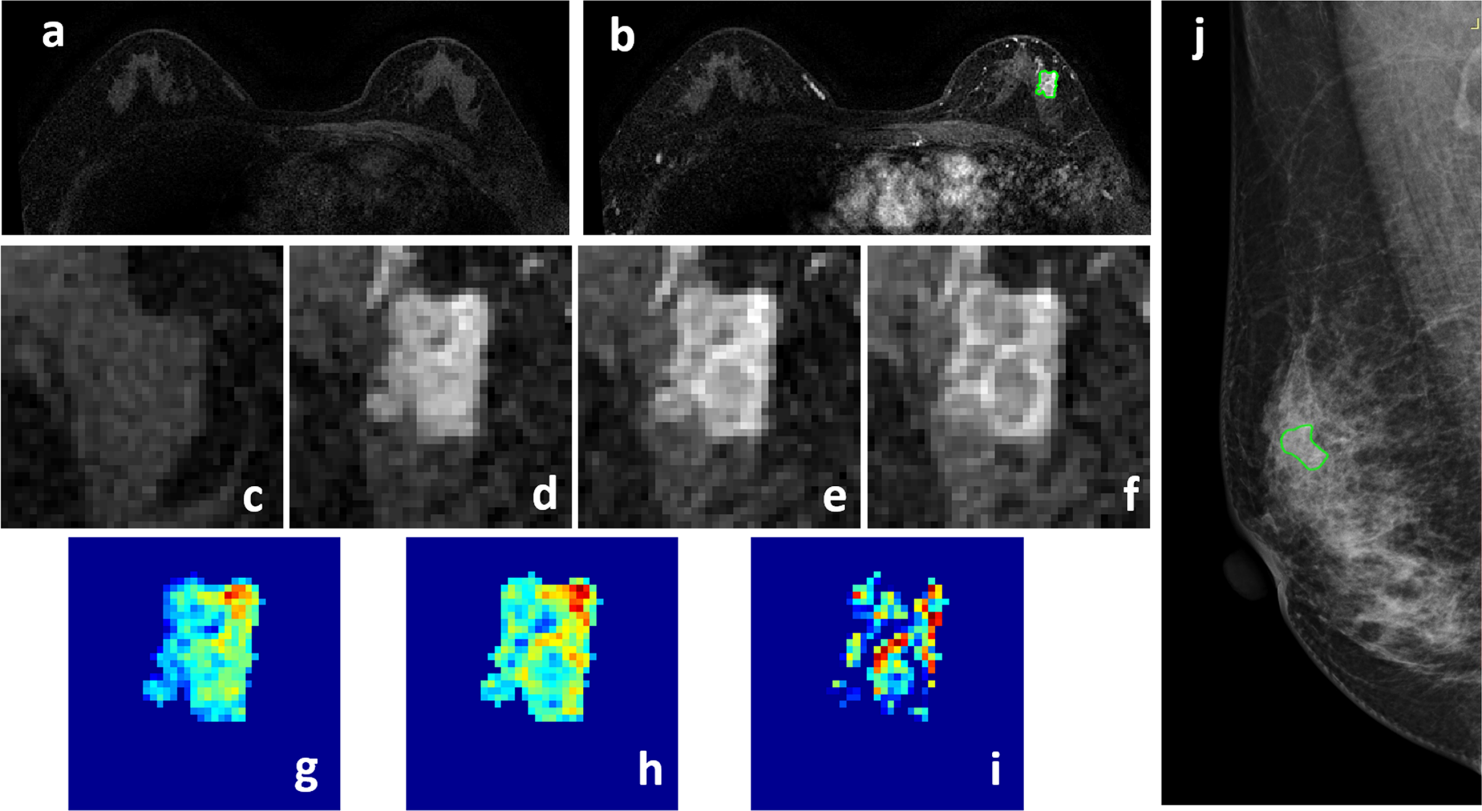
A 58-year-old patient with ductal carcinoma *in situ*, showing a strongly enhanced heterogeneous 1.4 x 0.9 cm lesion, with MRI BI-RADS score of 5. (A) F1 Pre-contrast image. (B) F2 post-contrast image. (C–I) Magnified images to demonstrate the margin and internal enhancements within the lesion. (C) F1 pre-contrast, (D) F2 post-contrast, (E) F3 post-contrast, (F) The last F6 post-contrast image. (G) The wash-in signal enhancement map F2-F1, (H) The F3-F1 signal enhancement map, (I) The wash-out F6-F3 map. (J) A suspicious BI-RADS 4A mass is seen on mammography. The lesion ROI is outlined with the guidance of MRI. The probability predicted by MRI, mammography, and combined radiomics models were: 0.53, 0.49, 0.62, respectively, true positive.
